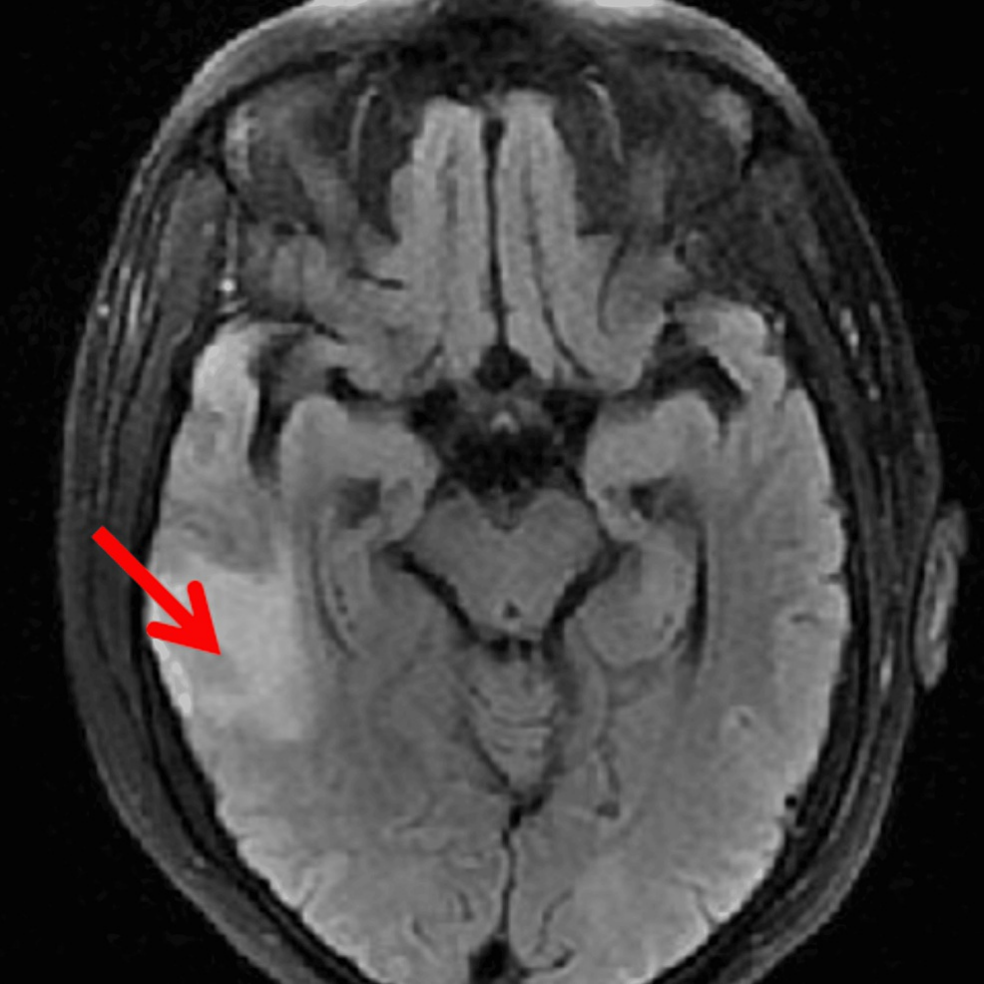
MRI showing cerebral infarction in the right temporal lobe (red arrow)
